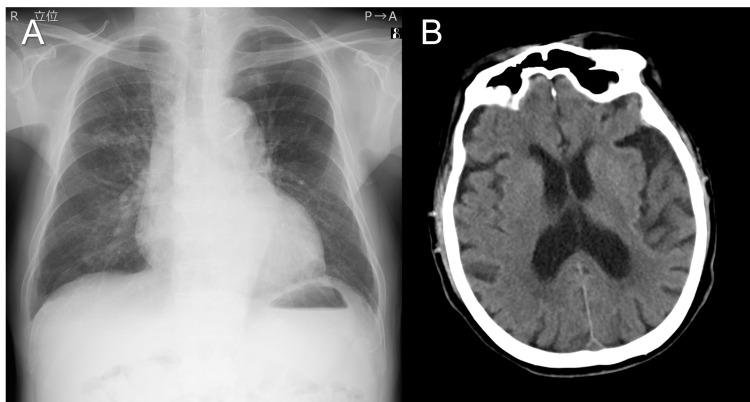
Imaging studies on admission. (A) The chest radiograph revealed no abnormalities. (B) The computed tomographic images of the brain showed no abnormalities.
